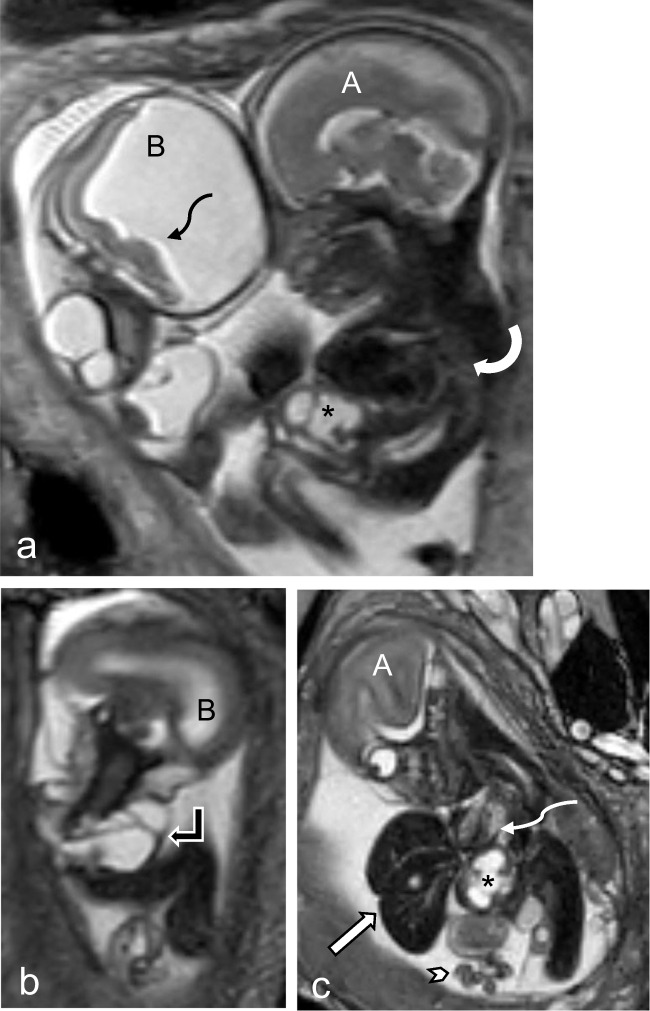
Fetal MRI of craniopagus at gestational age 24 weeks, 6 days, showing dorsal cephalic union, in addition to substantial individual congenital anomalies. This pair was joined only by a scalp skin bridge (confirmed at birth). a T2-weighted SSH TSE sagittal image to twin A’s brain shows separate intracranial compartments for twin A and twin B. Twin B demonstrates alobar holoprosencephaly (*curvy black arrow*), while twin A demonstrates a hypoplastic chest (*curved white arrow*) with partially imaged thoracoabdominoschisis and pelvicaliectasis (*black asterisk*). b BTFE image coronal to twin B’s body shows a terminal myelocystocele (*black right-angle arrow*). Twin B has a nonviable appearance. Twin B’s chest is small without candidates for heart or lung tissue. Additionally, there was no identified liver, spleen, kidneys, or bladder in twin B. c BTFE sagittal to twin A’s body shows thoracoabdominoschisis with liver evisceration (*white arrow*), bowel evisceration (*white arrowhead*), mild ectopia cordis (*curvy white arrow*), and pelvicaliectasis (*black asterisk*). Twin A also had evisceration of spleen and stomach with scoliosis and absence of the lumbosacral vertebral bodies with overall findings consistent with limb body wall complex. *BTFE*, balanced turbo-field echo; *SSH TSE*, single-shot turbo spin-echo
